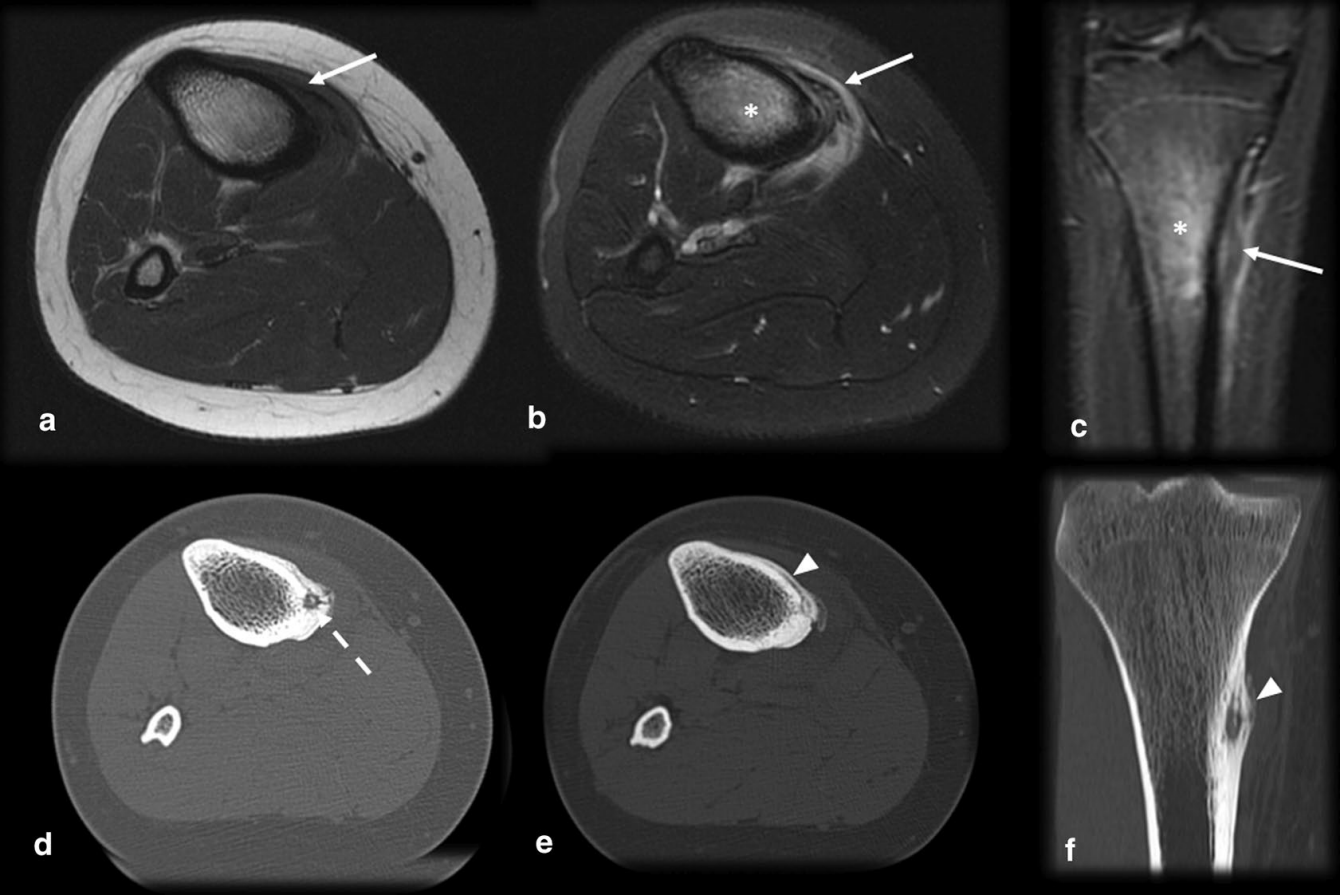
Osteoid osteoma versus tibial stress syndrome. A 15-year-old male soccer player with posteromedial tibial pain for 3 months that worsened while training and upon palpation of the upper posteromedial tibia. MRI axial T1 (a), axial (b) and coronal (c) T2 FS showed cortical thickening, periosteal reaction, pes anserinus tendon edema (arrows) and bone marrow edema (asterisk), mimicking a stress syndrome. Further investigation with a CT scan (d, e, f) demonstrated a nidus (dashed arrow), causing this corticoperiosteal reaction (arrowhead) and confirming OO
